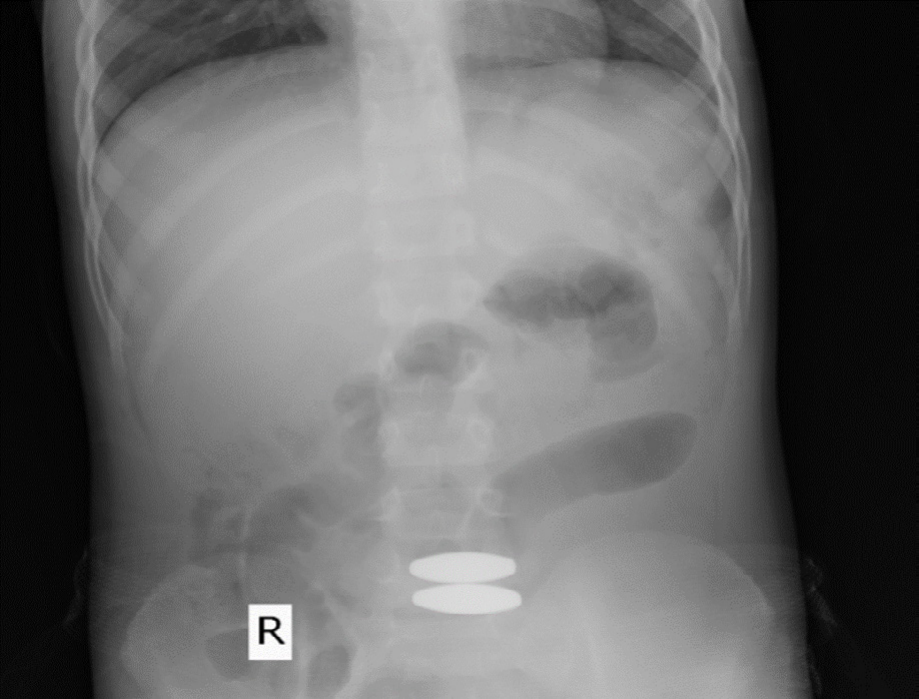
X-ray abdomen showing free gas under the ameasuring ~2 x 1 cm.
